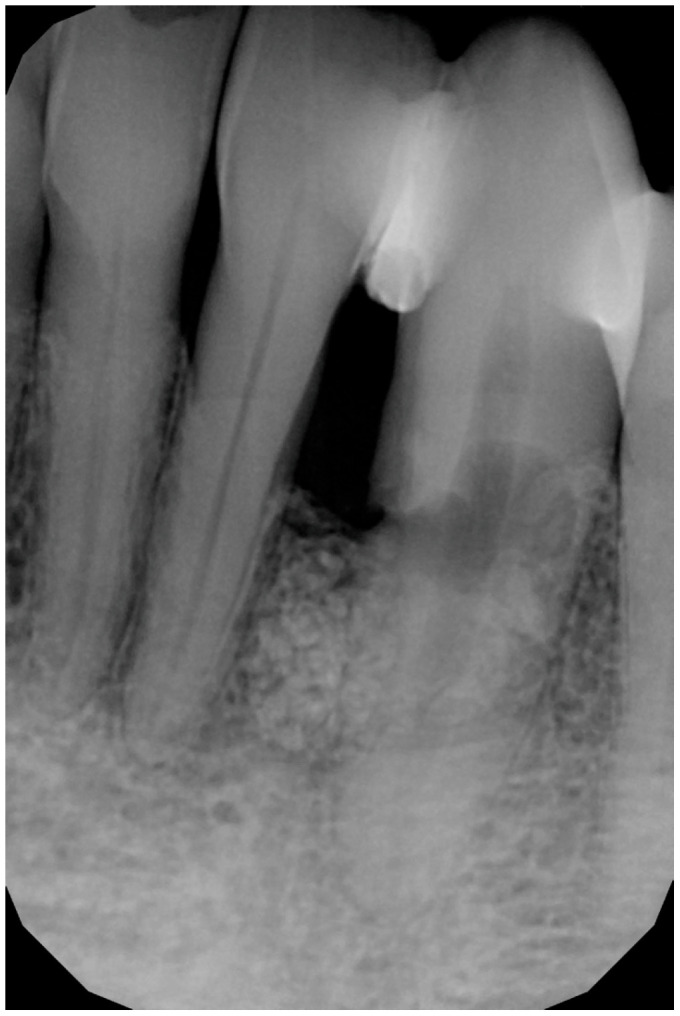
Intraoral X-ray at baseline, highlighting the damaged root of element 33.
